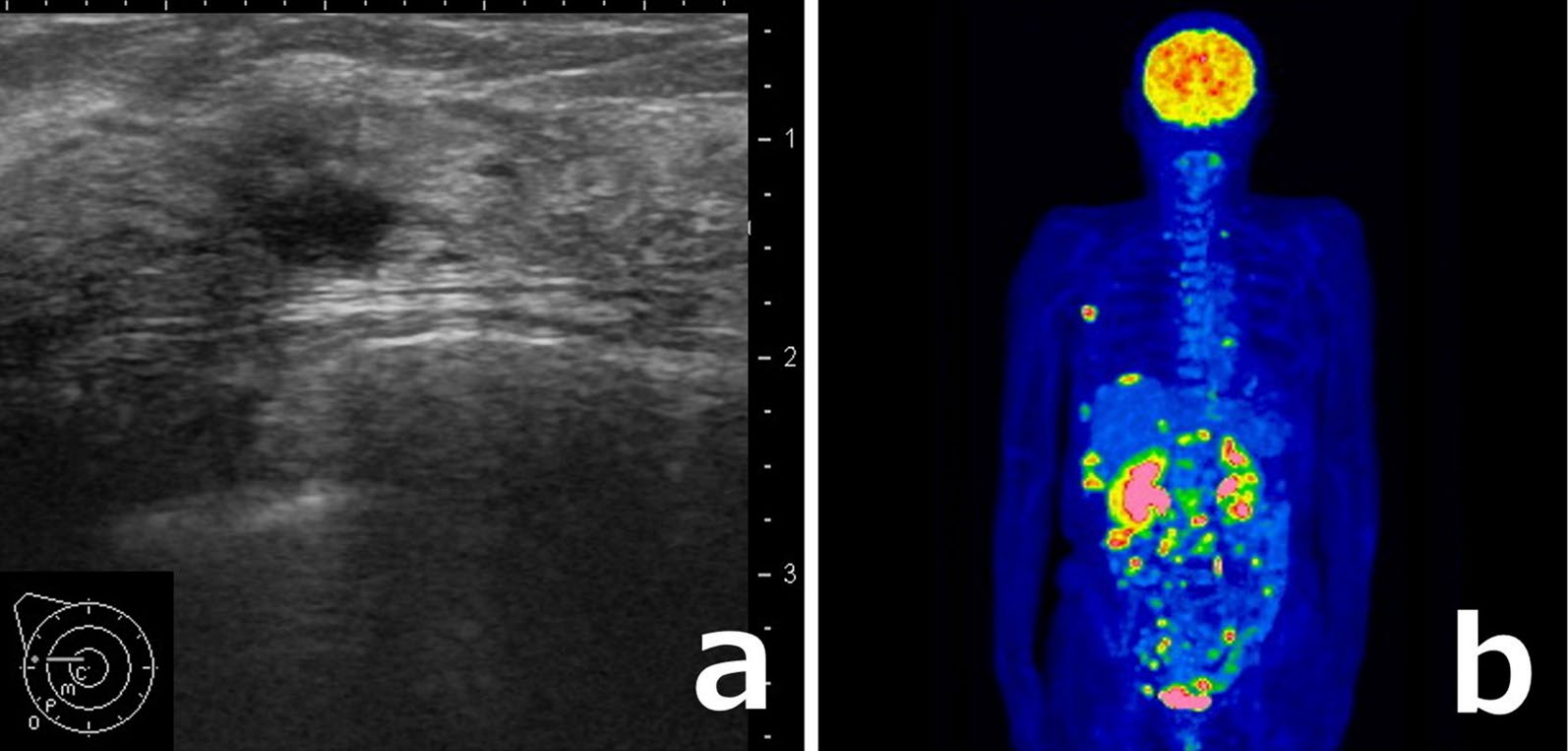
Case 1: imaging findings. a Ultrasound revealed a low-echo area of approximately 5 mm in the upper-outer quadrant of the right breast. b Positron emission tomography–computed tomography showed numerous foci of dissemination and lymphadenopathy in the abdominal cavity, including the intestine directly under the artificial anus, the right lumbar subcutaneous region, and the right uterine appendages
